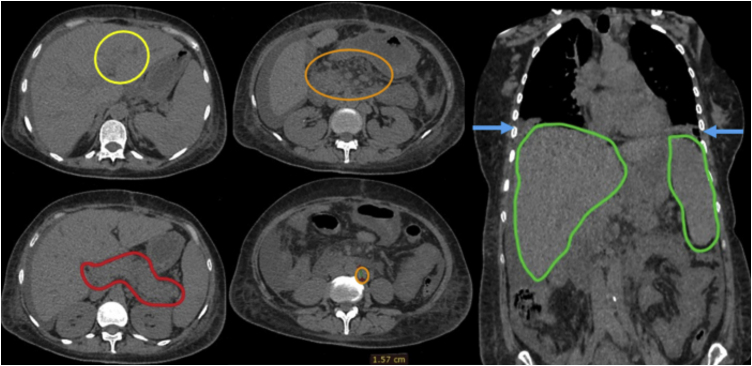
Computed tomography of the abdomen; yellow circle: intrahepatic bile duct ectasia; red circle: pancreas of heterogeneous volume and density; orange circles: enlarged intra-abdominal lymph nodes; blue arrows: bilateral pleural effusion; green circles: hepatosplenomegaly.
